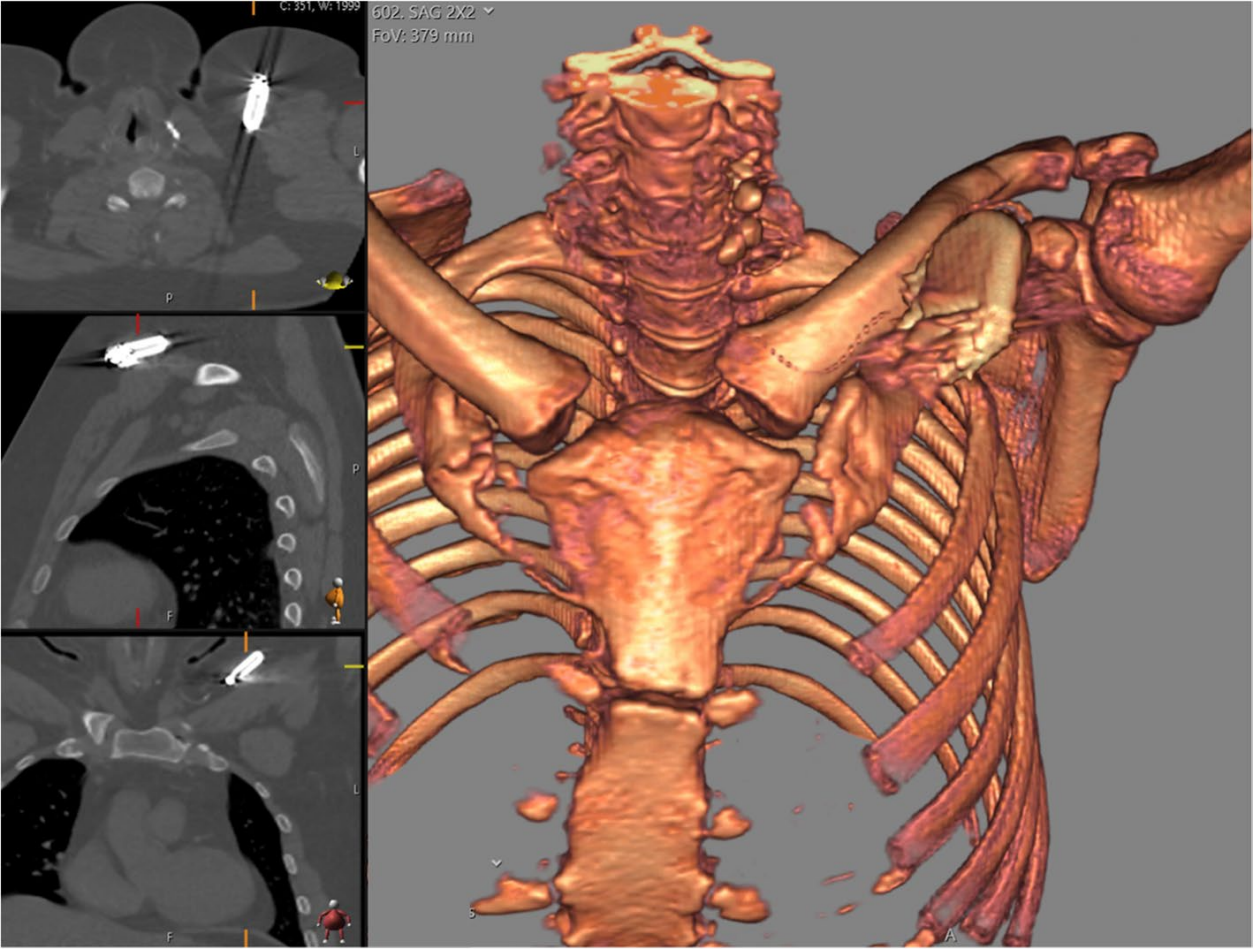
Preoperative CT of the neck and chest demonstrating oblique positioning of IPG without other VNS hardware or surrounding tissue abnormalities
